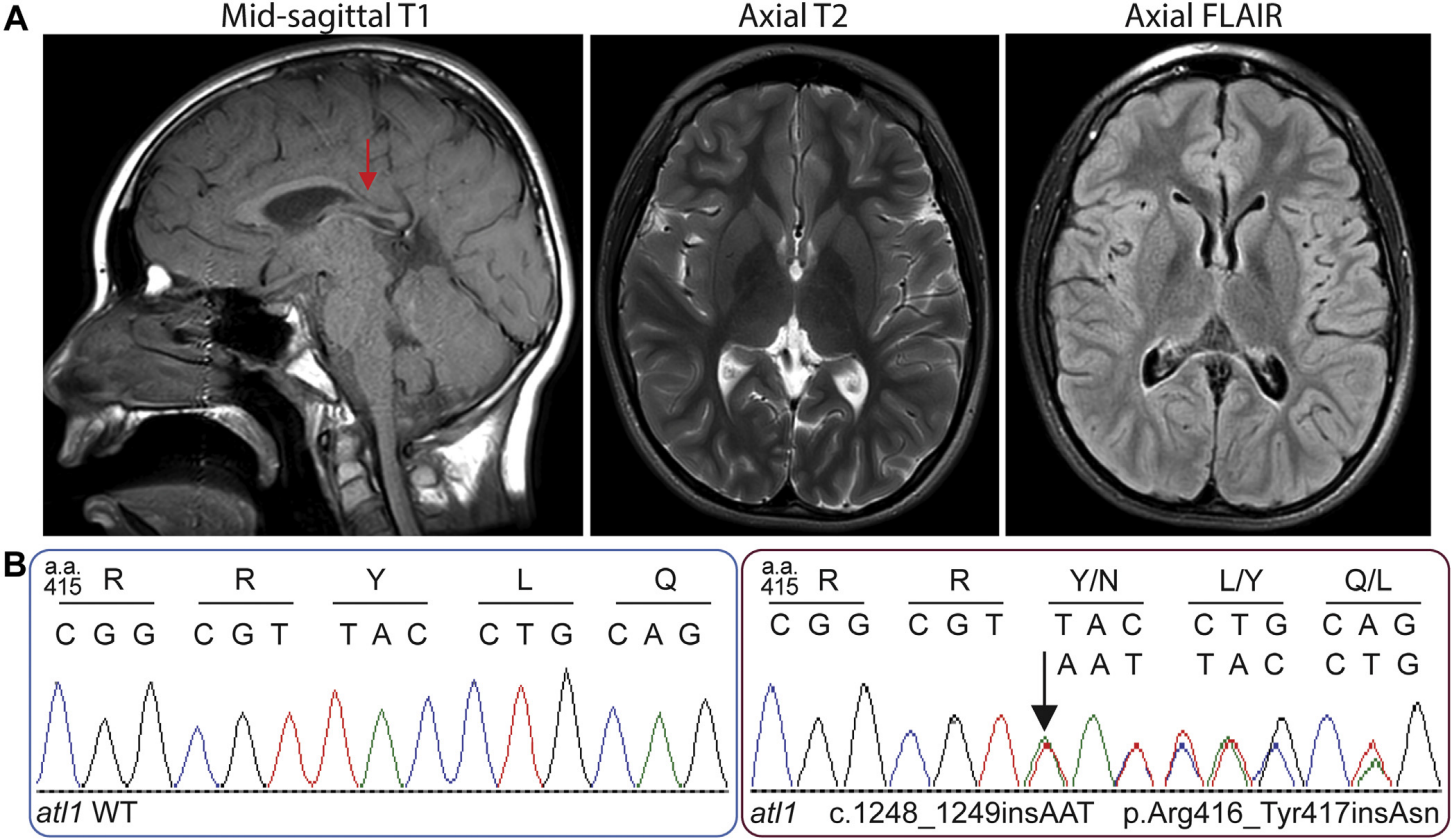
MRI and Sanger sequencing of proband.*A*, MRI at 15 years showing thinning and down-sloping of the posterior body of the corpus callosum (*arrow* in *left panel*). *B*, Sanger sequencing chromatograms of *atl1* WT (*left*) and mutant (*right*) from the proband, starting at position 415 (amino acid)/1243 (nucleotide). The *arrow* indicates the location of the inserted codon (AAT) between position 1248 and 1249. Single letter amino acid and nucleotide codes indicated above.
